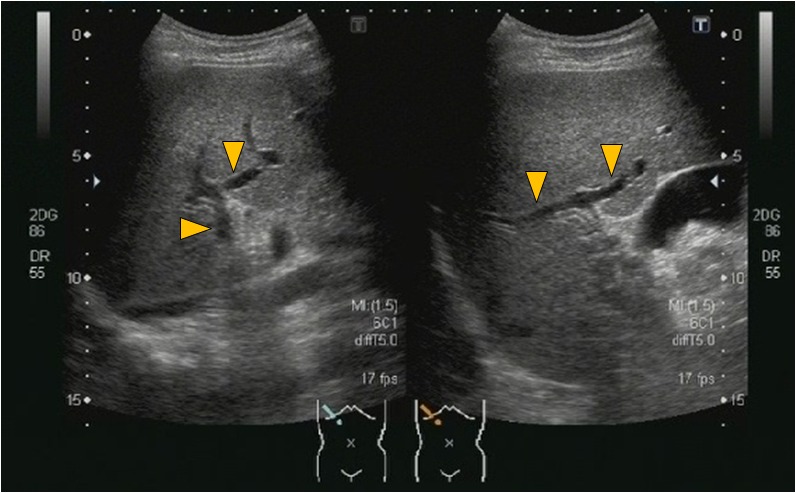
Abdominal ultrasonography (US) findings on hospital day 22. B mode US images show that the thrombus remaining in the right main branch of the portal vein had been mostly dissolved (arrowheads).
